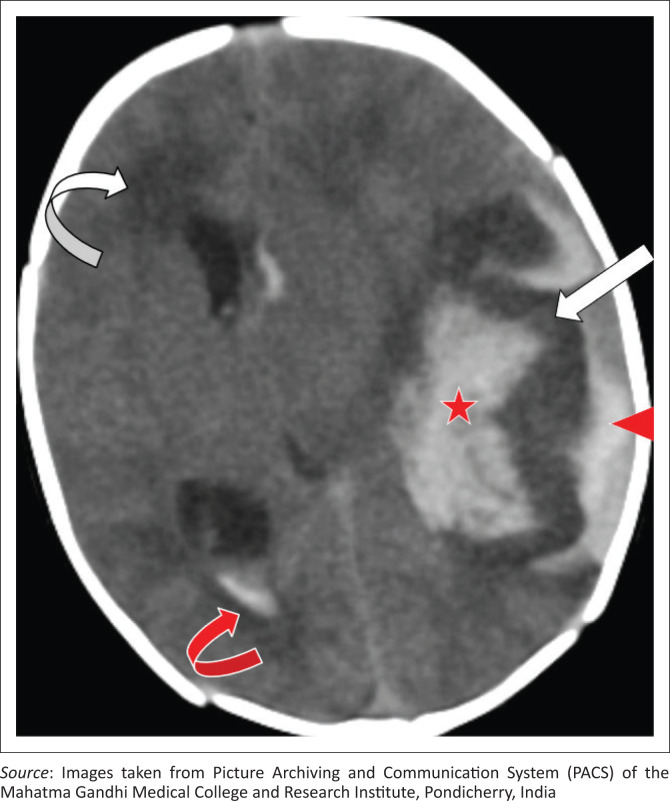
Axial CT brain. Localised extra-axial hyperdensity in the left temporal region (red arrowhead) and left temporal lobe parenchymal hyperdensity (red star) representing subpial haemorrhage with intraparenchymal extension. The cortical hypodensity (white arrow) represents ischaemia. Blood products also identified in the ventricular system (red curved arrow) with hydrocephalus and transependymal oedema (white curved arrow).
